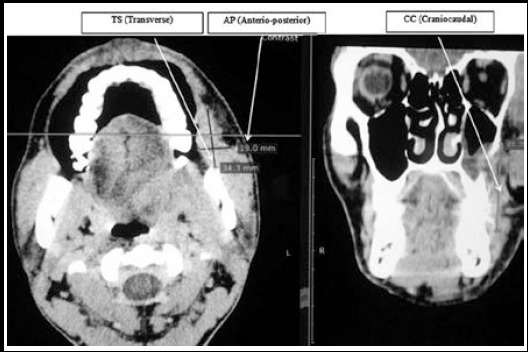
Dimensions of TS, AP and CC as marked on CT Scan Neck with contrast.
